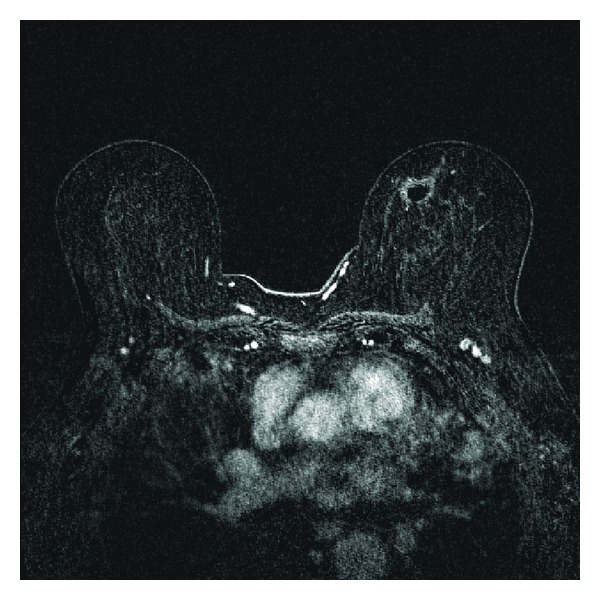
Subtraction MRI demonstrates a 2.1 cm × 1.2 cm enhancing mass within the lingula, biopsy proven to represent extranodal Castleman's disease. Incidental note is made of a postbiopsy hematoma/seroma within the medial left breast from stereotactic biopsy of the pleomorphic calcifications demonstrated in Figures 1(a) and 1(b).
